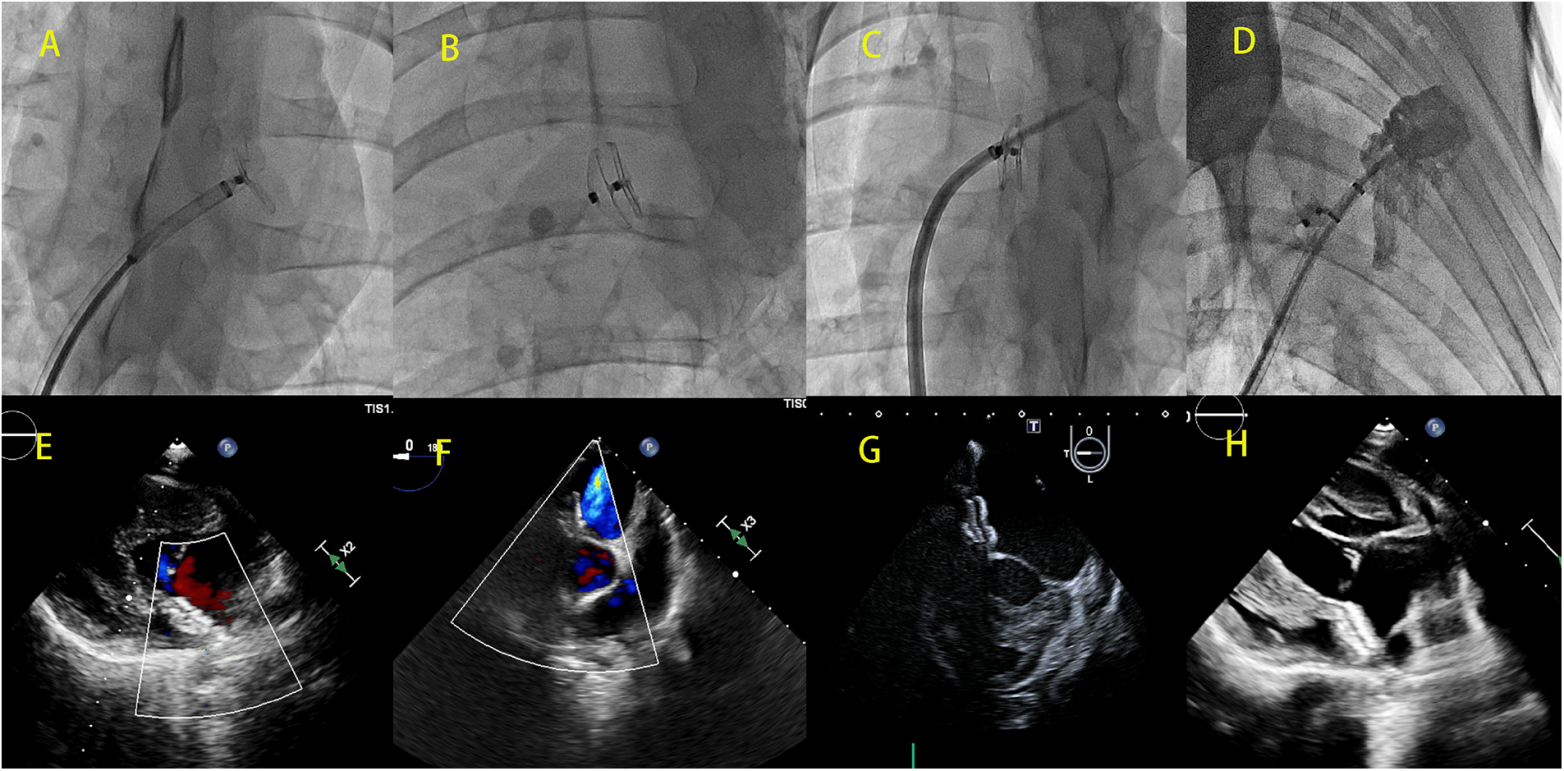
DSA images (A–D) and cardiac ultrasound images (E–H) showing the implantation of a bilateral asymmetric single-rivet PFO occluder device in the reserved interatrial septum puncture area. (A) Left ventricular disc of the deployed occluder device; (B) morphology of the fully deployed occluder device; (C) successful puncture of the interatrial septum through the puncture site reserved by the occluder device after 3 months of initial implantation; (D) left atrial angiography performed through the sheath inserted via the interatrial septum; (E,F) no residual shunting observed immediately after the implantation of the occluder device; (G,H) cardiac ultrasound demonstrating the morphology of the occluder device after implantation.
